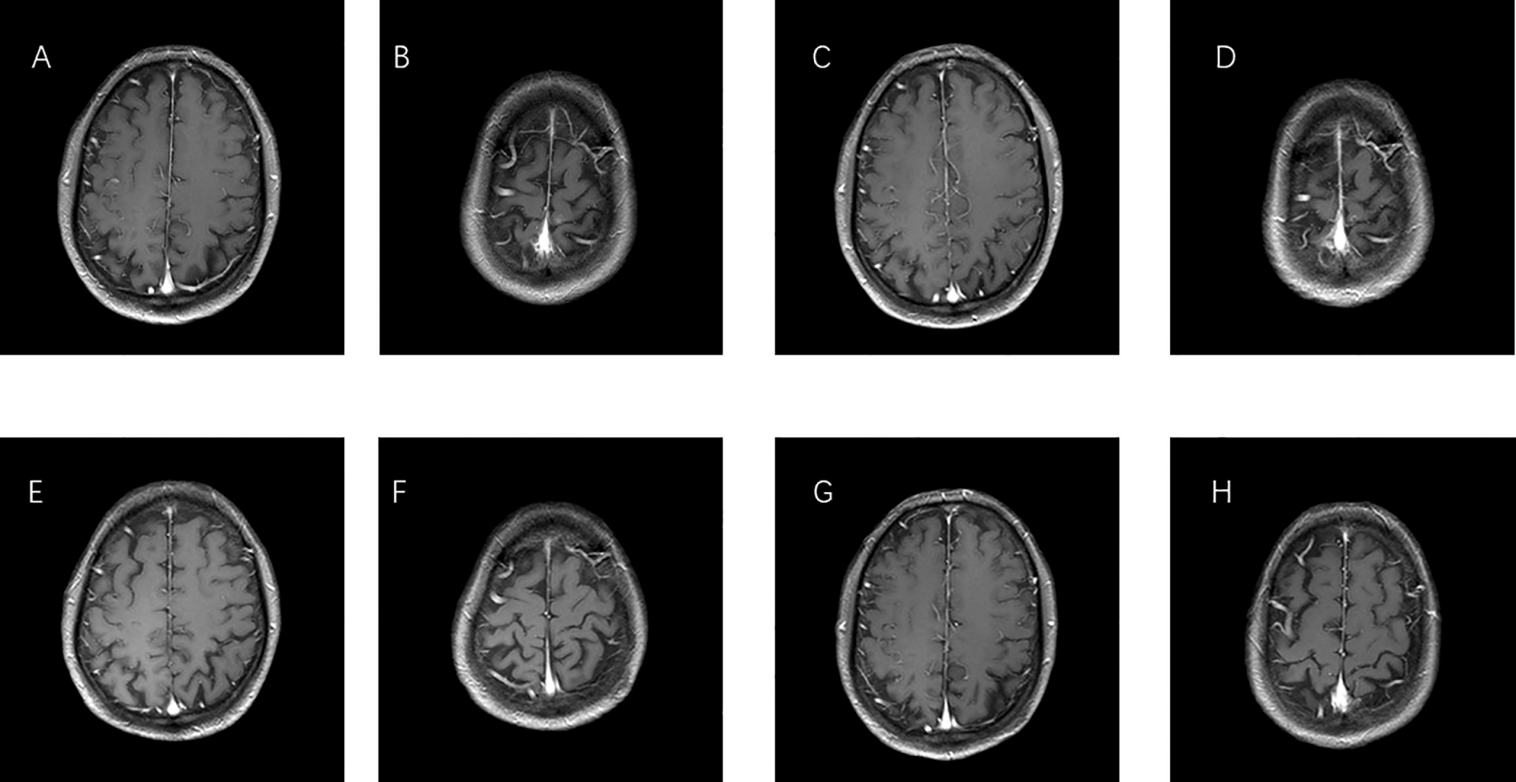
MRI images of brain metastases. (A, B) Before therapy, imaging performed on 30 December 2016. (C, D) One year after taking gefitinib on 19 March 2018. (E, F) One year after taking Osimertinib on 18 February 2019. (G, H) Last check on 20 May 2022.
